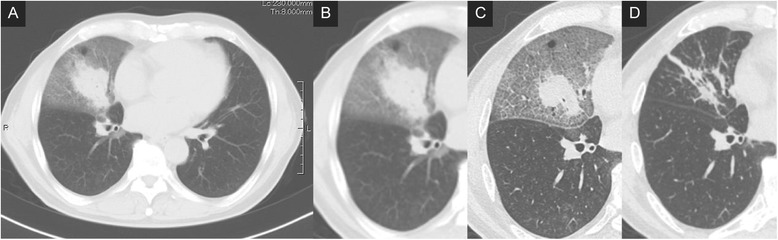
Serial chest computed tomography images of a 66-year-old male with lipoid pneumonia. Ground-glass opacities with interlobular septal thickening (crazy-paving pattern) and partial infiltration in the right middle and bilateral lower lobes were observed on admission (a, b). The infiltrative shadow showed slight improvement after steroid pulse therapy (c). Subsequent bronchoalveolar lavage resulted in significant improvement (d)
